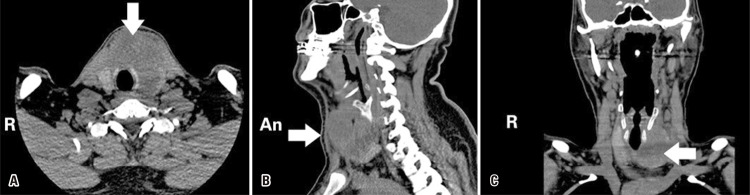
Axial (A), sagittal (B) and coronal (C) non-contrast computed tomography images of the patient. There is a heterogeneous, hypodense lesion located on the left lobe through the isthmus level, and the necrotic-fluid density areas extending from the midline to the right lobe (white arrow) An: anterior; R: right side.
